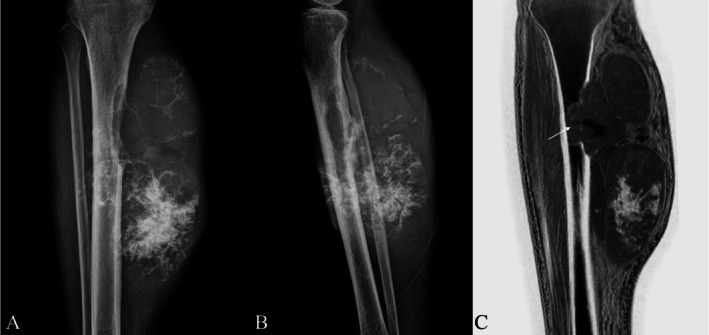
Anteroposterior (A) and Lateral (B) radiographs of the right tibia showing a large expansile mass with rings‐and‐arc type calcification with extraosseous soft‐tissue component arising from the diaphysis. Corresponding FRACTURE sequence (C) clearly demonstrates the intramedullary extent of the mass (white arrow).
